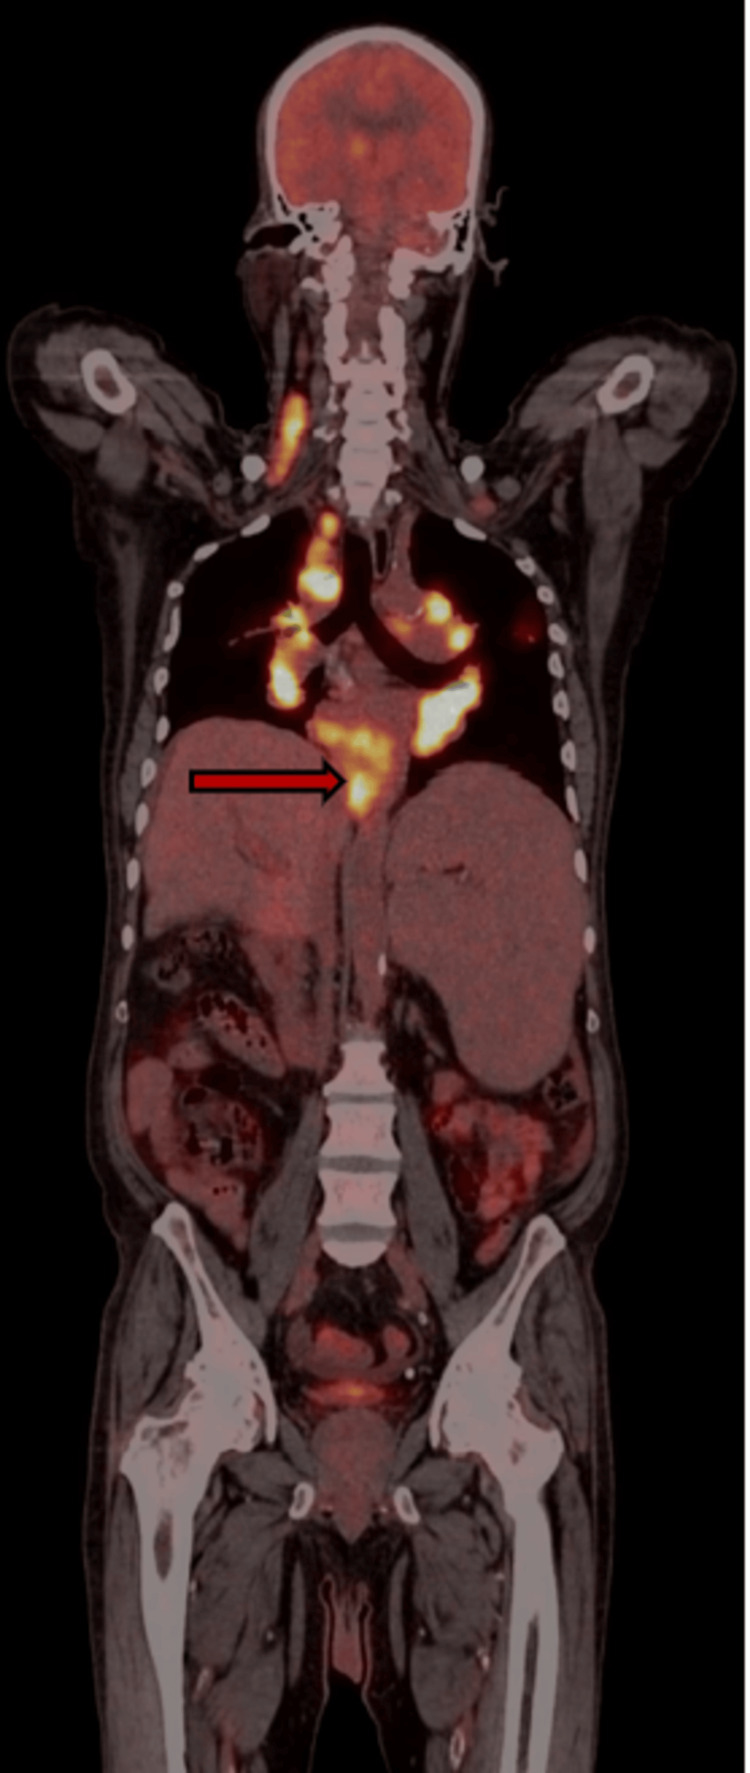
Coronal fused ¹⁸F-fluorodeoxyglucose (FDG) positron emission tomography (PET)-computed tomography (CT) image showing hypermetabolic lymphadenopathy involving paraesophageal nodal stations as shown by the red arrow No focal FDG-avid lesions suggestive of a primary malignant mass are identified.
